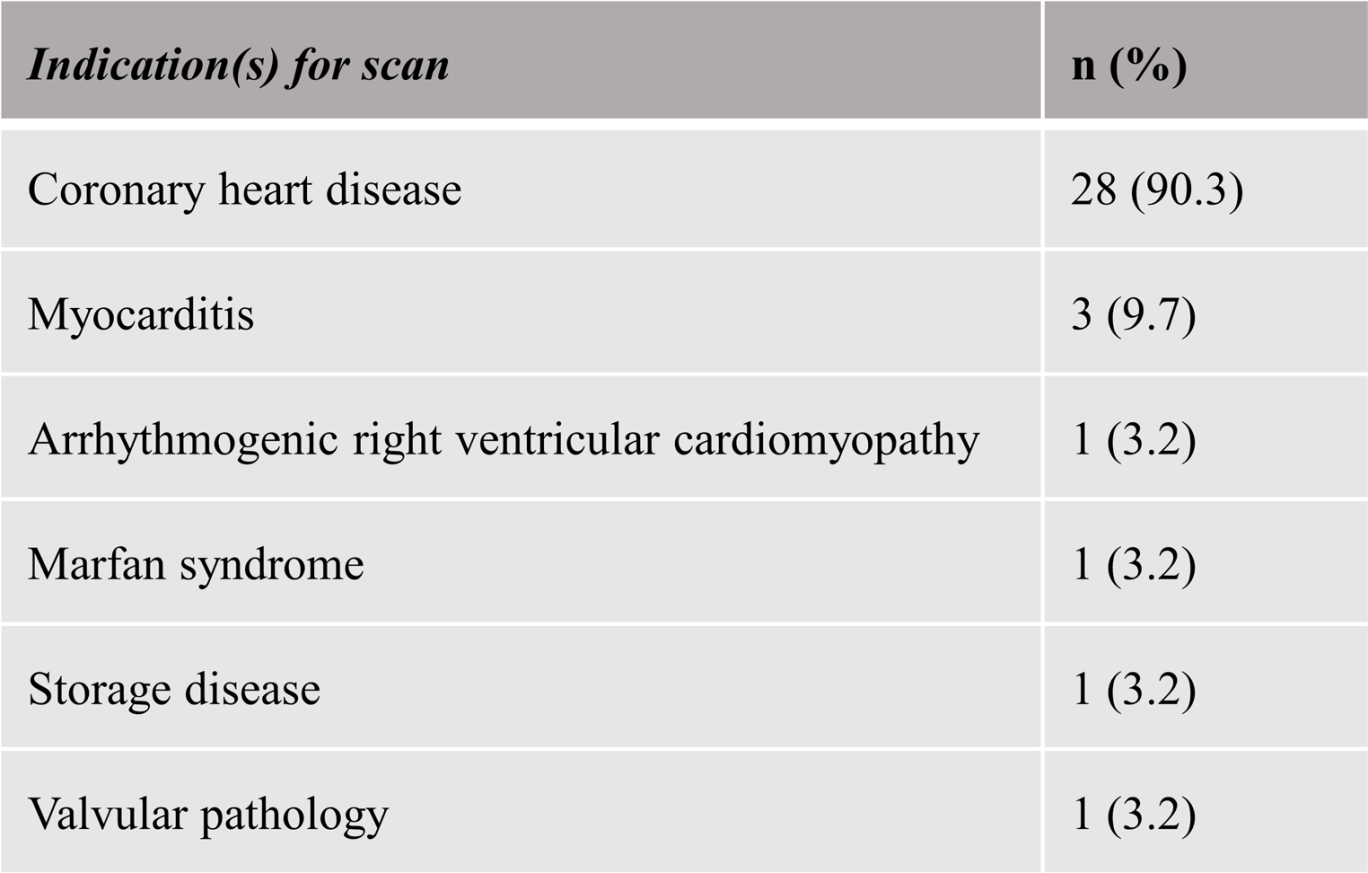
Indication(s) for scan. The indications for cardiac MRI included ischemic and non-ischemic cardiomyopathies. The number of clinical indications (n = 35) exceeds the patient number due to multiple indications in three cases
